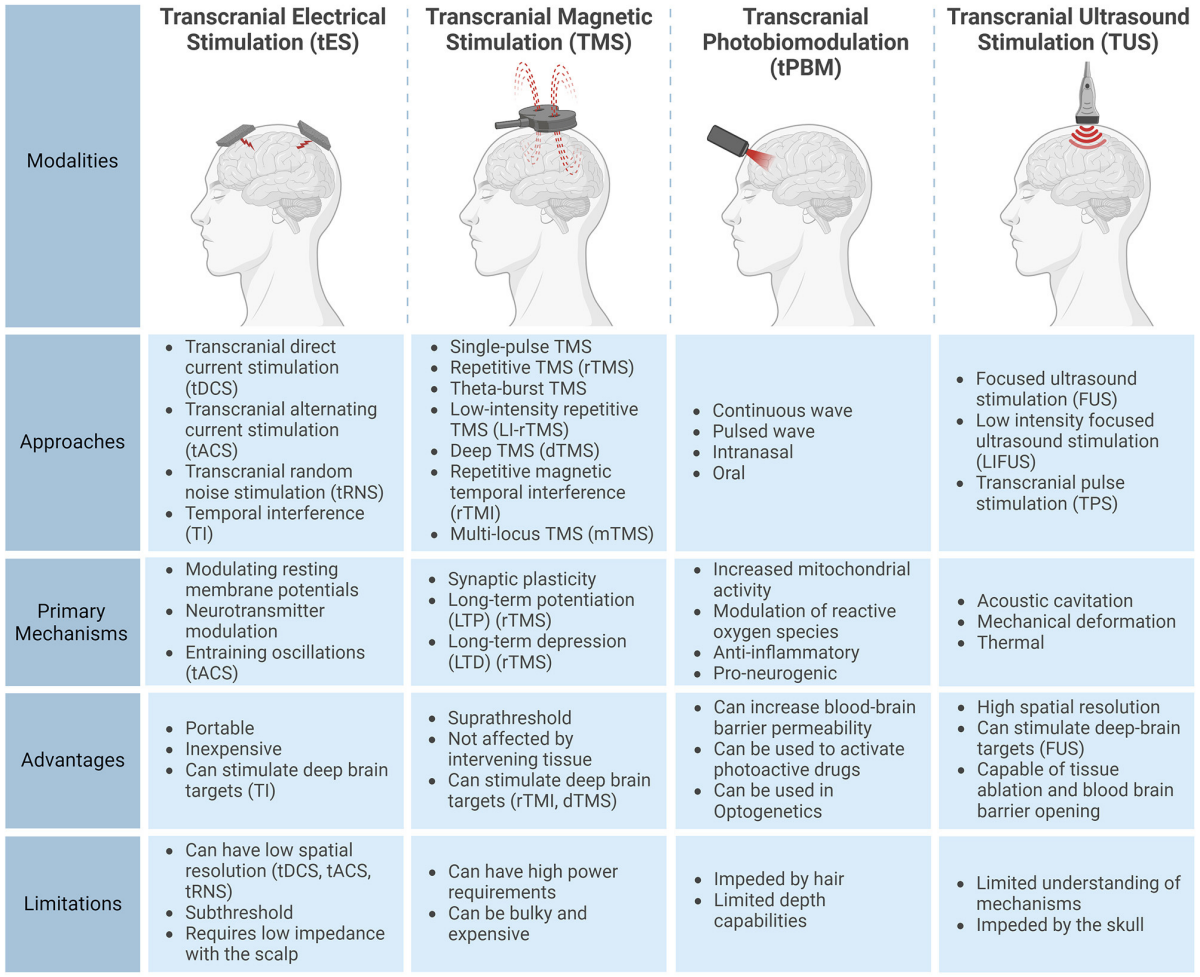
Characteristics of the four non-invasive brain stimulation modalities: transcranial electrical stimulation (tES), transcranial magnetic stimulation (TMS), transcranial photobiomodulation (tPBM), and transcranial ultrasound stimulation (TUS). This figure outlines common subtypes, primary mechanisms, advantages, and limitations for each modality. Created with BioRender.com.
